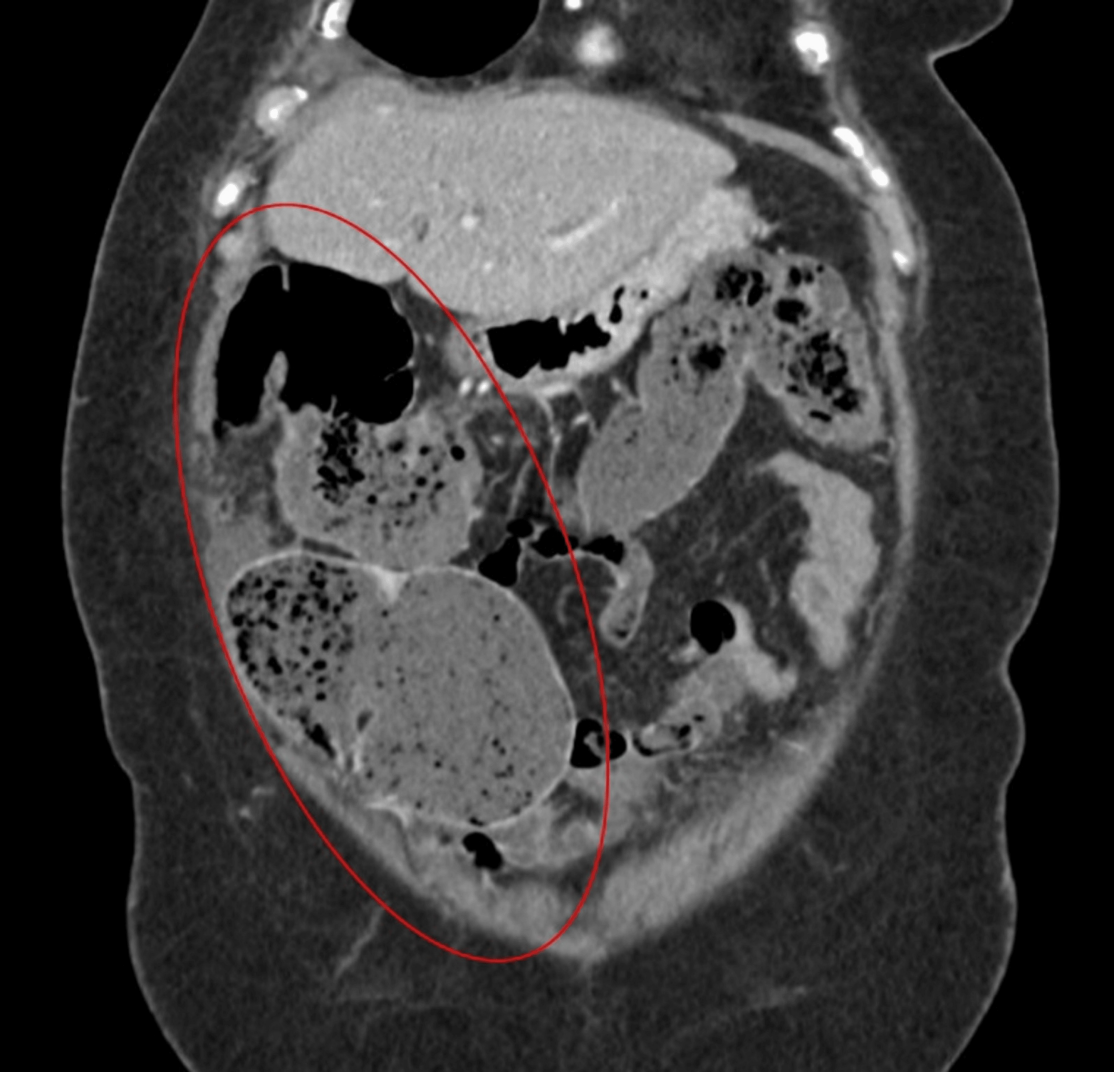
Abdomino-pelvic CT (coronal view) demonstrating cecal volvulus (red oval)
